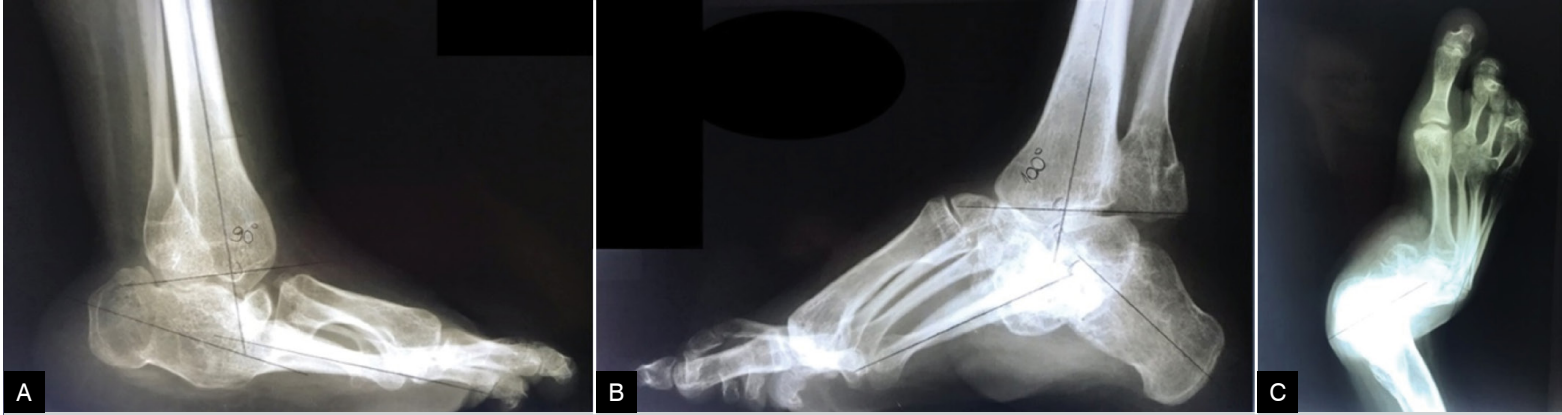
A) Lateral radiographic of the foot showing abnormal position due to talectomy in childhood, but the foot is plantigrade. B) Lateral alignment of a foot after talectomy with important cavus. C) Anteroposterior radiographic of a foot with lateral translation of the foot and adductus of the forefoot.
